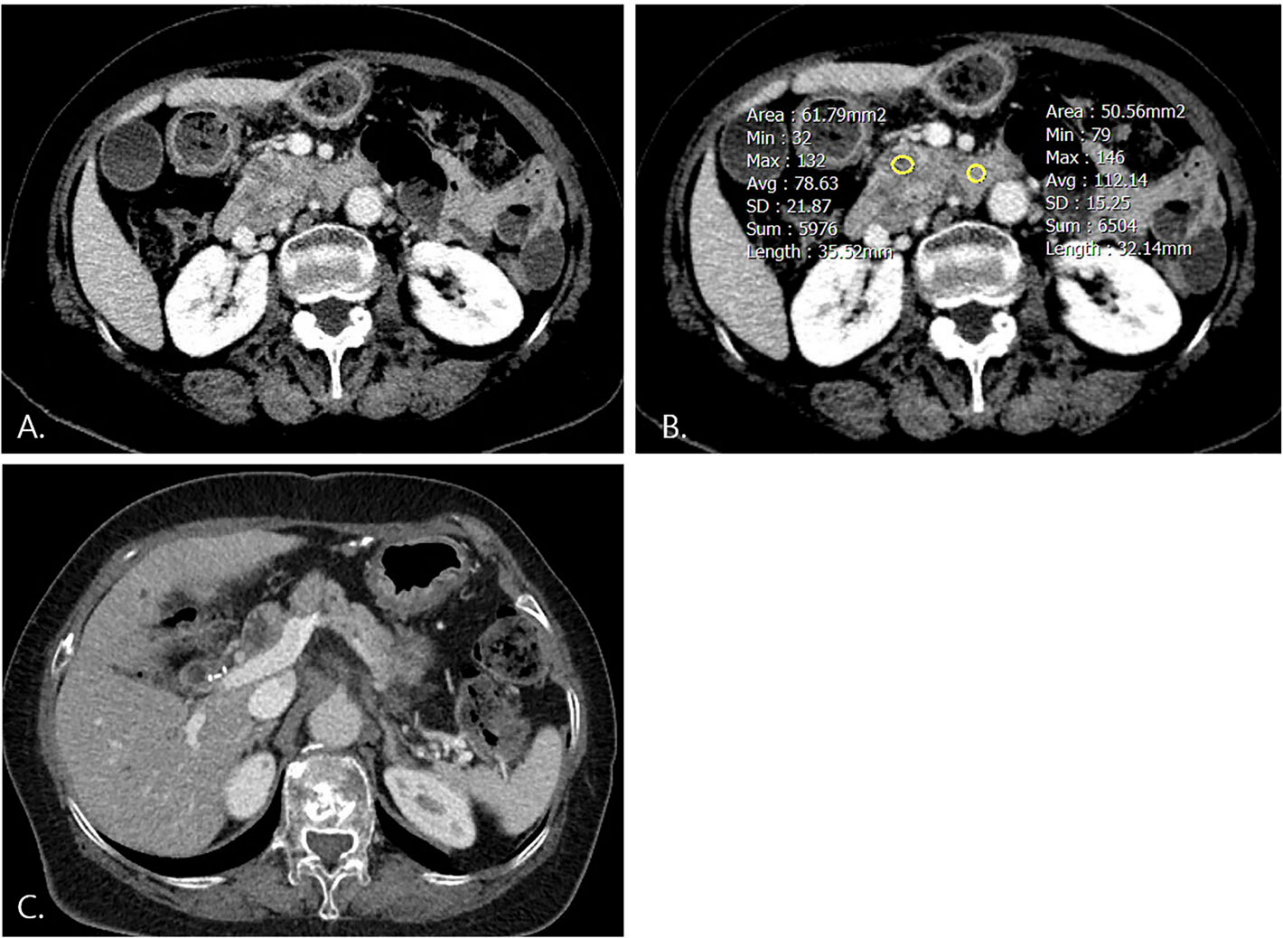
A 74-year-old female with resectable pancreatic cancer: conspicuity score < 2. a. Approximately 2.7-cm-sized hypovascular mass with low lesion contrast was visible in the pancreatic head on arterial phase contrast-enhanced CT. b. Conspicuity score was 1.42, preoperative CA 19–9 was 1466.33, and postoperative CA 19–9 was 7.88. c. There was no postoperative recurrence, and she is alive at 23 months after operation
